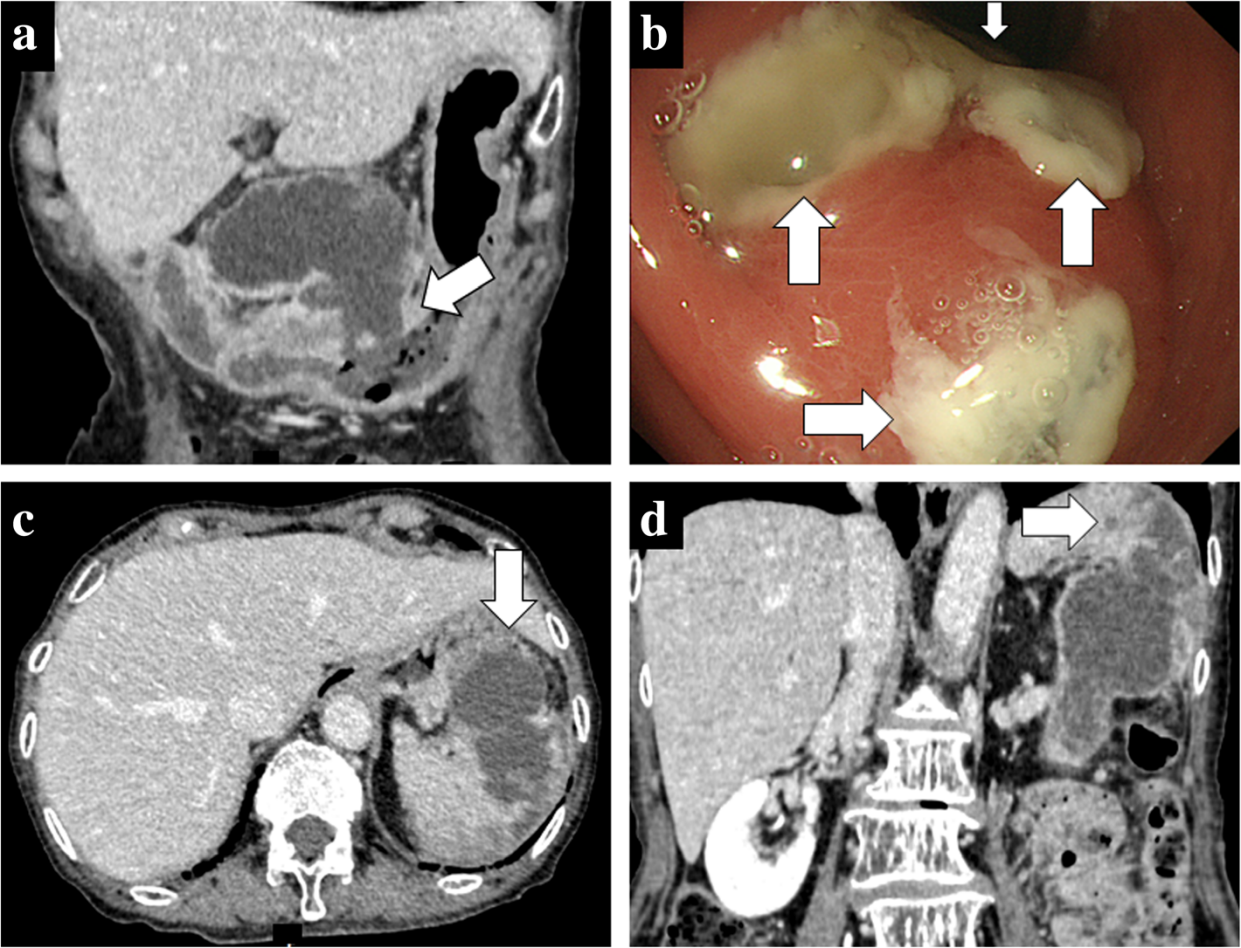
Gastropancreatic fistula and splenopancreatic fistula. a Contrast-enhanced computed tomography (CT) revealed fistulas (white arrow) between the pancreas and stomach. b Upper gastrointestinal endoscopy revealed four gastropancreatic fistulas on the posterior wall of the gastric body (four white arrows) and mucus discharge from the gastropancreatic fistulas. c, d Contrast-enhanced CT revealed fistulas (white arrows) between the pancreas and spleen
